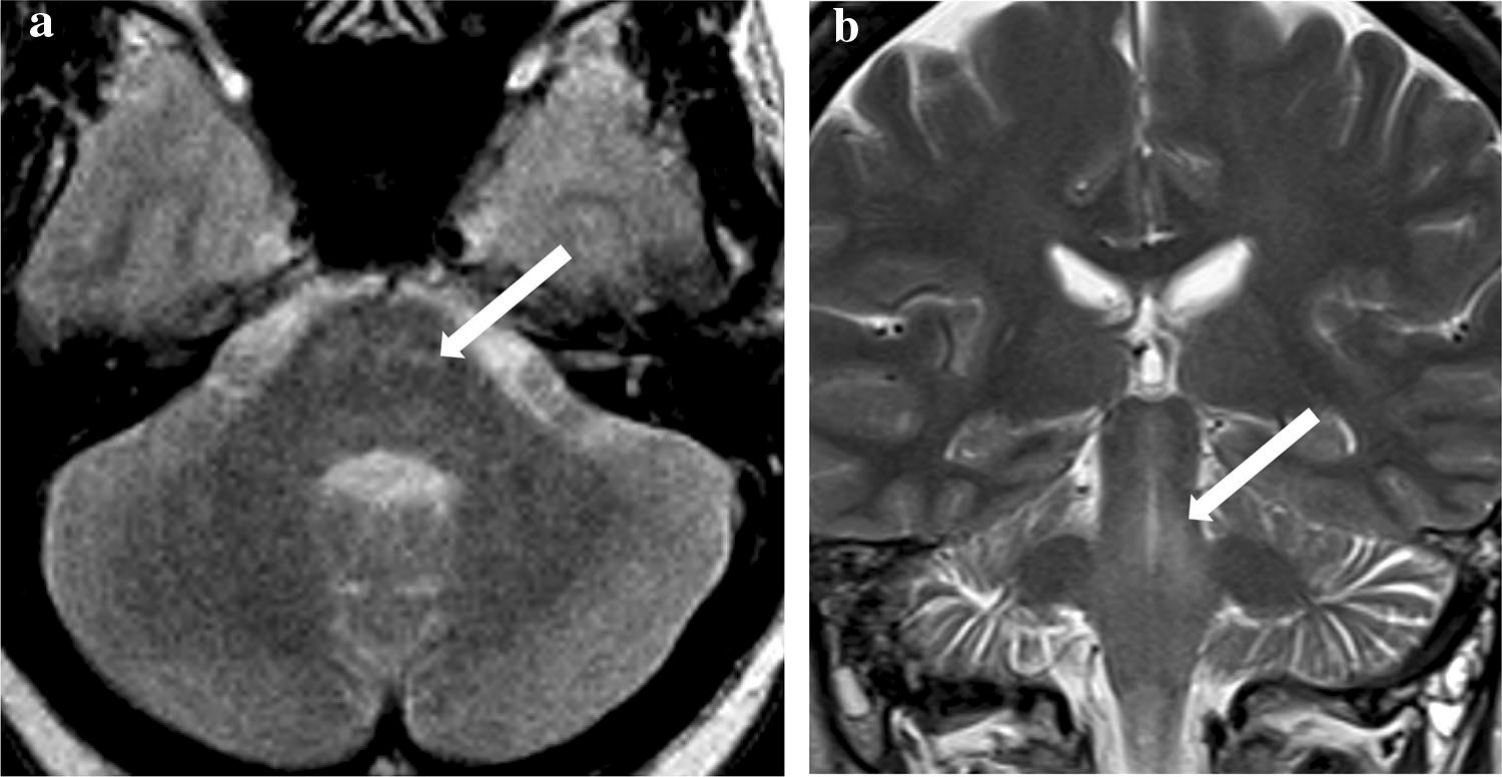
a Patient C MRI brain during third episode of HTLV encephalitis. Ill-defined T2w signal hyperintensity in the basis pontis. b Patient C brain MRI brain during fourth episode of HTLV encephalitis (while on ciclosporin). Coronal T2w sequence. Ill-defined T2 signal hyperintensity in the midbrain and pontine tegmentum. No swelling or pathological enhancement. Previous area of signal change now normalised
